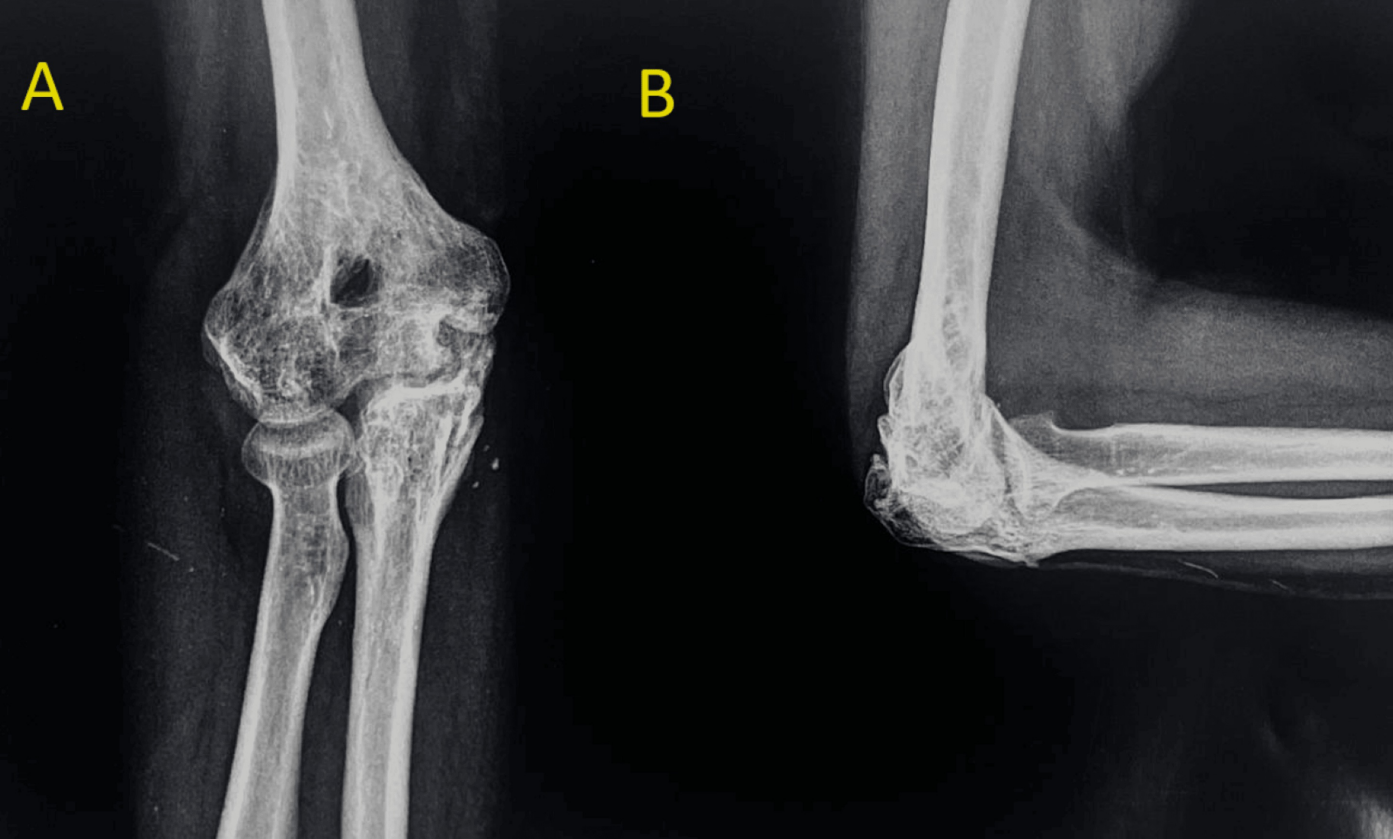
Follow-up radiograph after four years: (A) anteroposterior view and (B) lateral view
